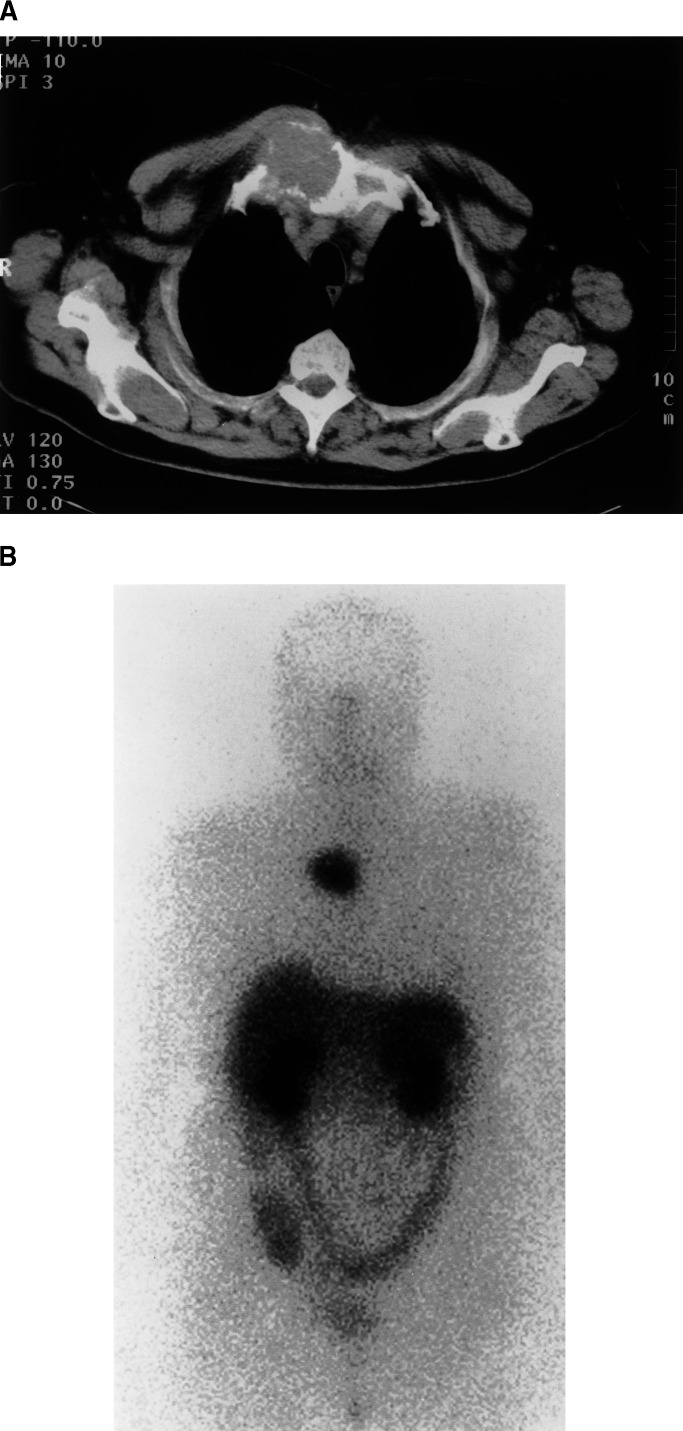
Comparative images of a patient with an isolated sternal metastasis secondary to a Hurthle cell thyroid primary tumour. Image (A) is cross-sectional CT slice through the deposit. Image (B) is the ^111^In-DTPA^0^octreotide scan showing intense uptake at the site of the deposit.
